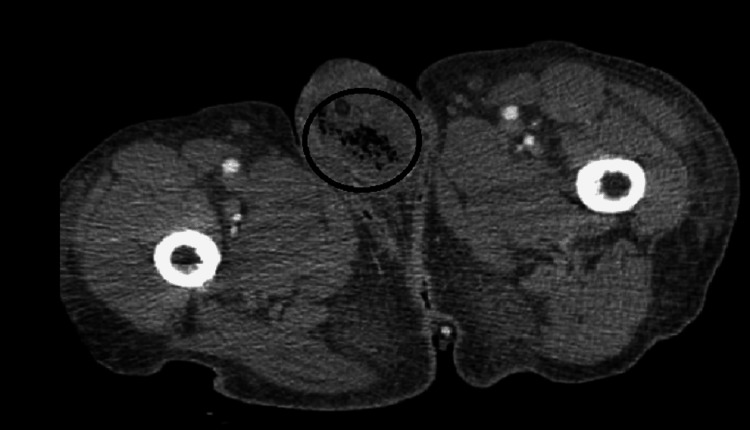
A computed tomography scan of the abdomen and pelvis on day 5 of admission revealed gas in the anterior penis
